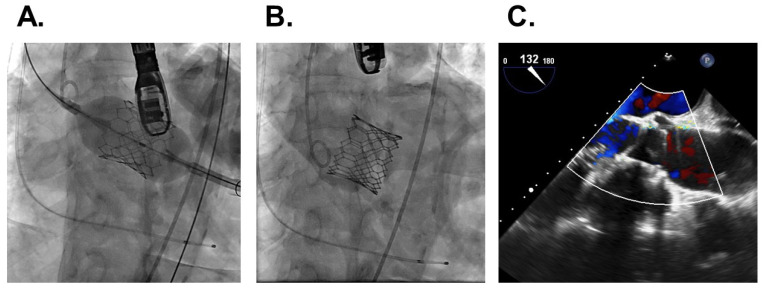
Trans-apical transcatheter aortic valve replacement. Aortic valve implantation (A), aortography without any peri-valvular leak (B), and transesophageal echocardiography displaying no peri-valvular leak (C).
